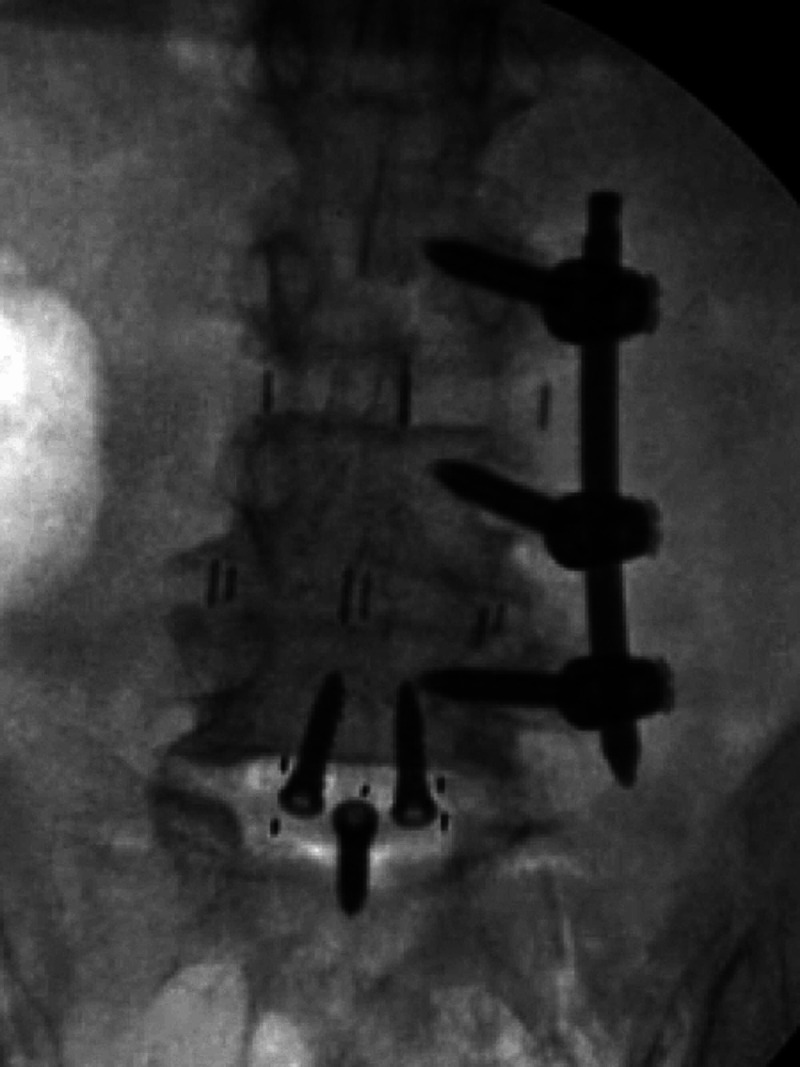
Anterior-posterior X-ray following placement of the L5-S1 anterior interbody demonstrating unilateral posterior instrumentation from L3-L5 on the left side
